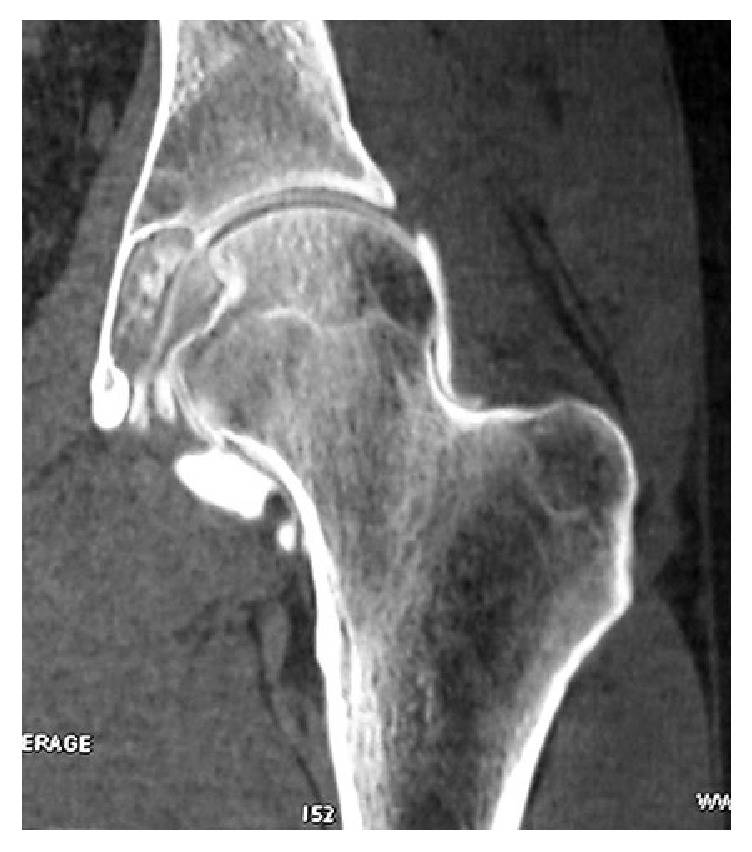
CT arthrogram revealing a lesion expanding medially through the acetabular fossa with sclerotic rim.
